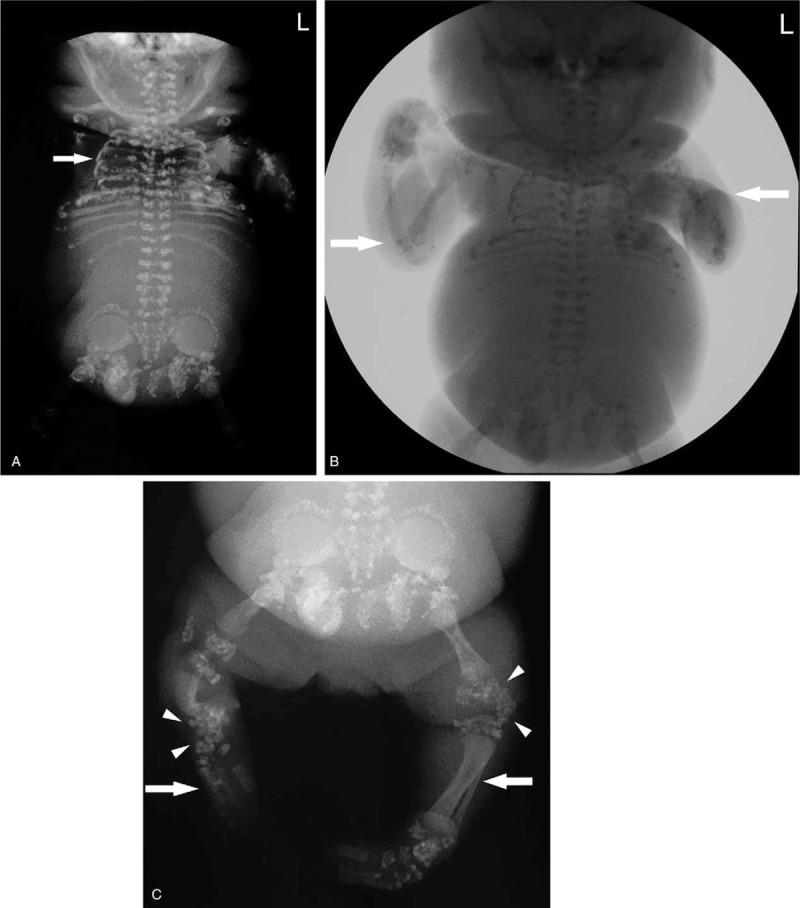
Post-delivery X-ray: (A) small and narrow thorax (arrows); (B) short limbs (arrows); and (C) asymmetric lower limbs (arrows), punctate changes in the metaphysic (arrowheads).
